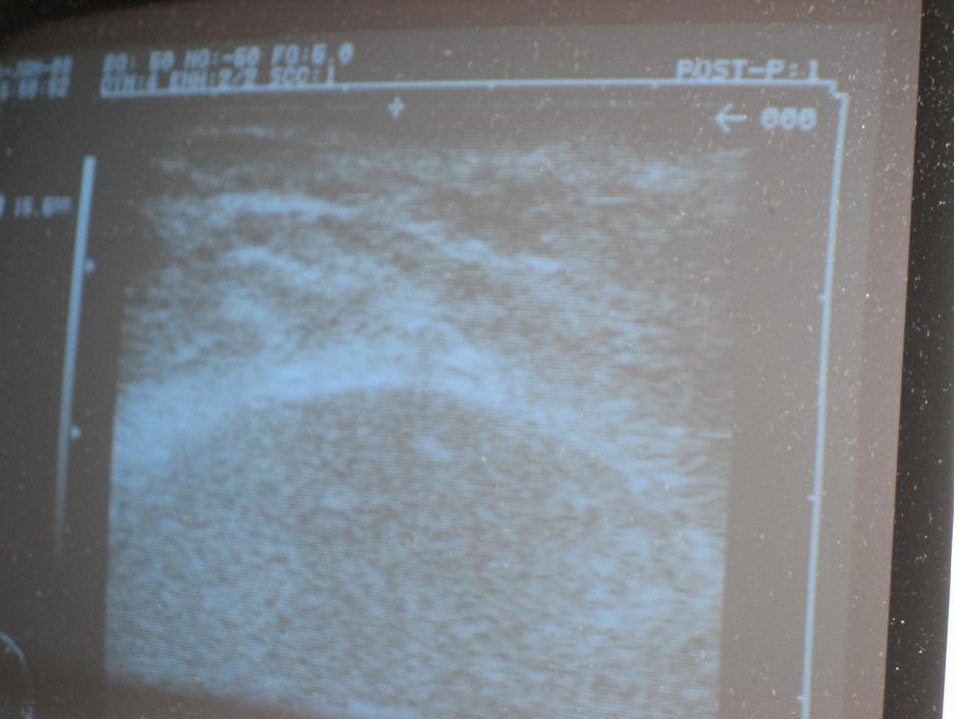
Ultrasound image showing a balloon-skin-distance of 1.6-cm and no evidence of air pockets surrounding the balloon.
